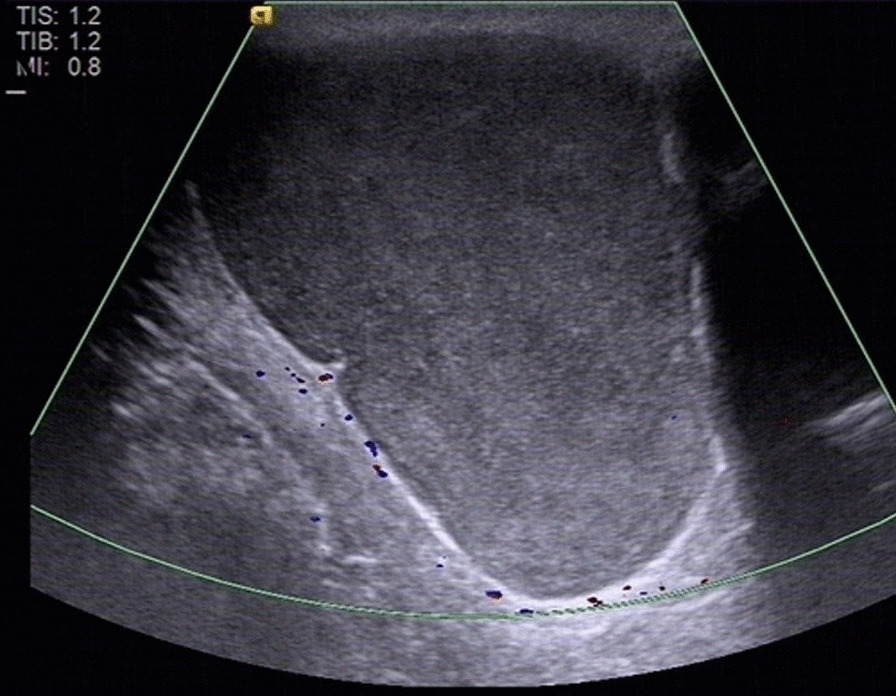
Ultrasonography shows a hypoechoic lesion with internal echogenic foci and posterior acoustic enhancement
